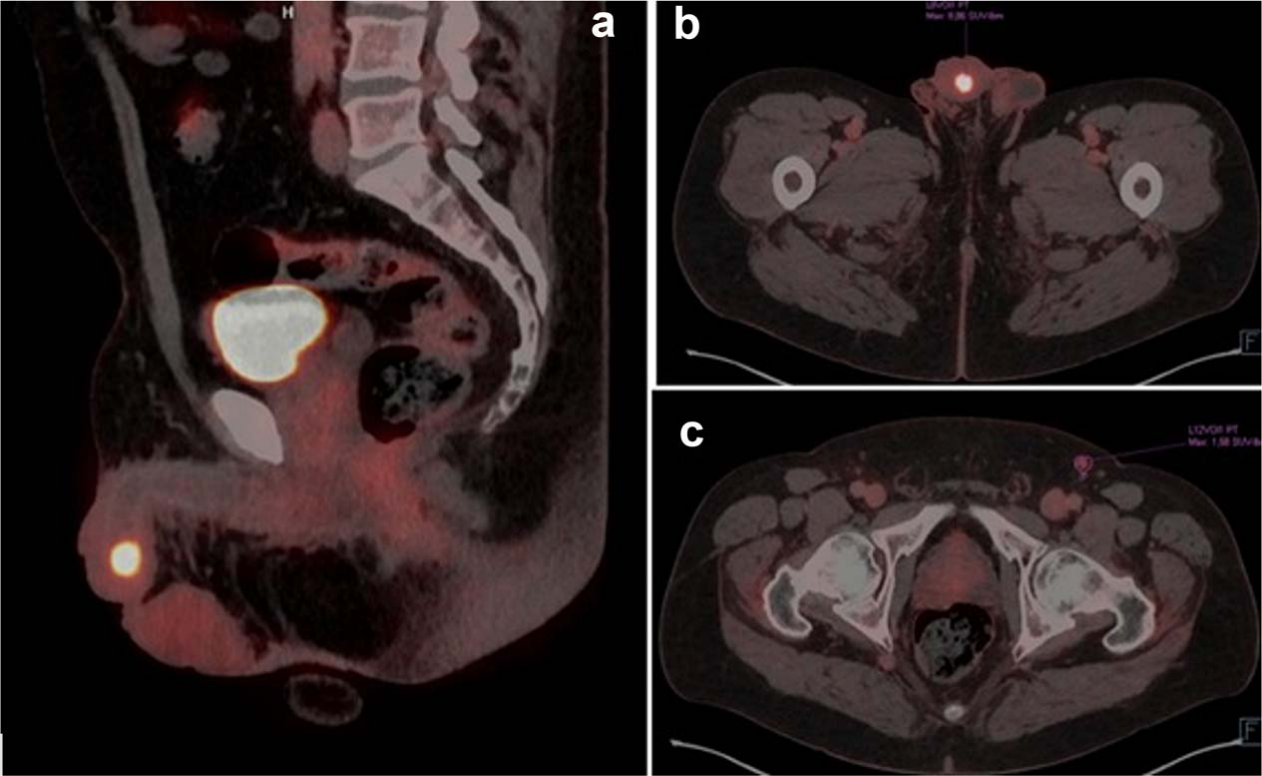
(a and b) PET-TC FDG-18 showing hypermetabolic lesion in glans. (c) Left inguinal lymph node positive in PET-TC.
